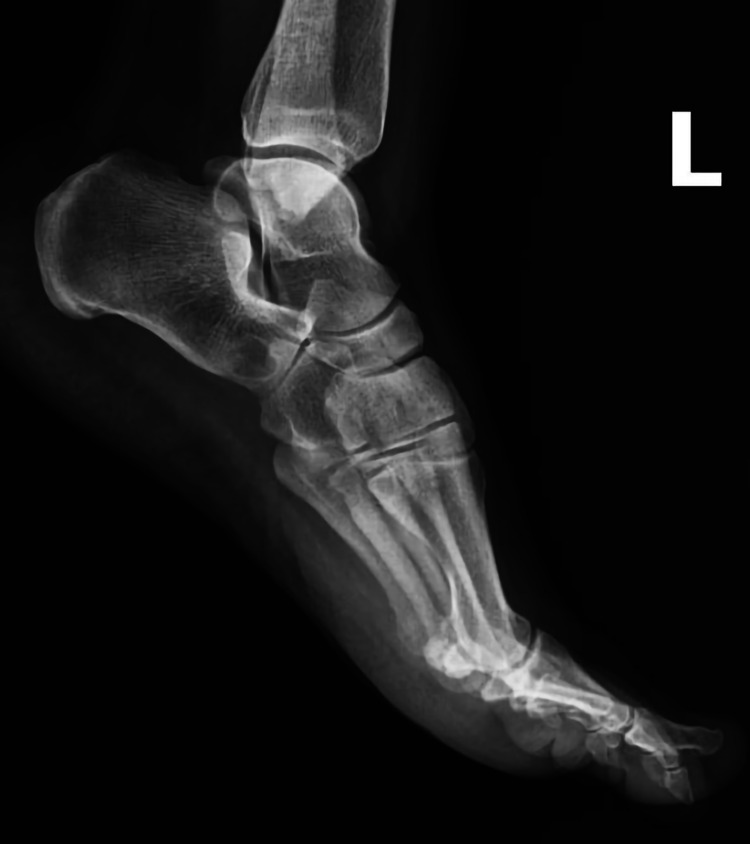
Preoperative lateral foot X-ray image
